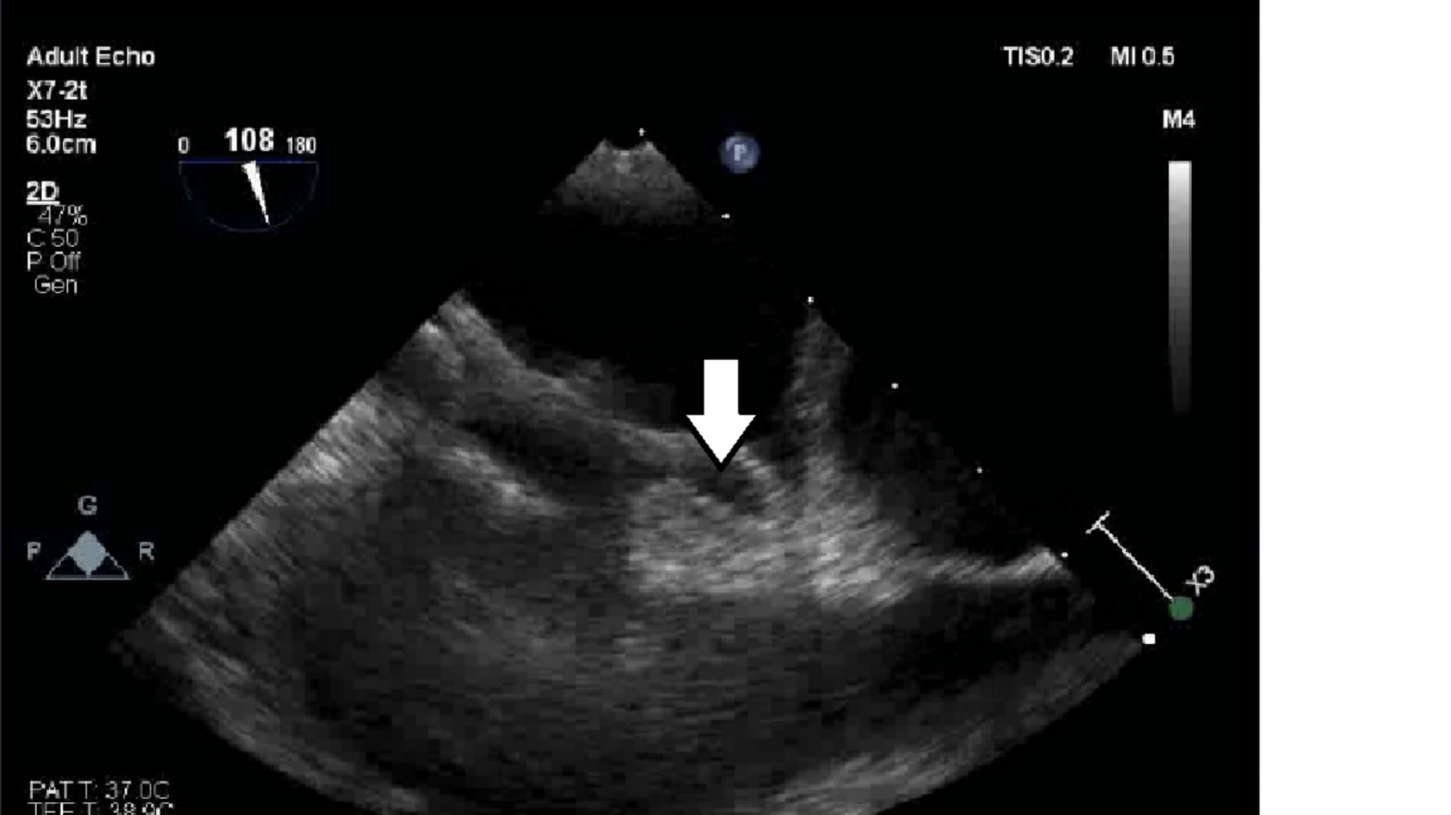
Transesophageal echocardiography showing #28 Amplatzer septal occluder device (arrow)
